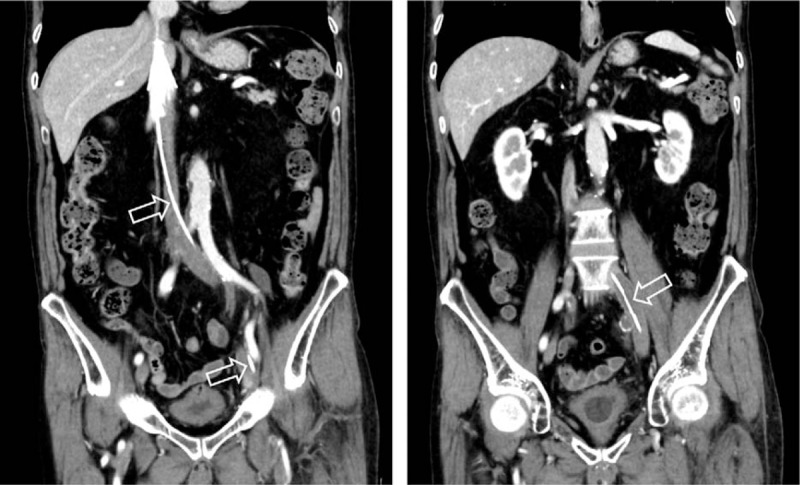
Abdominal computed tomography scan showing catheter-directed thrombolysis treatment using a Fountain infusion catheter (arrow).
